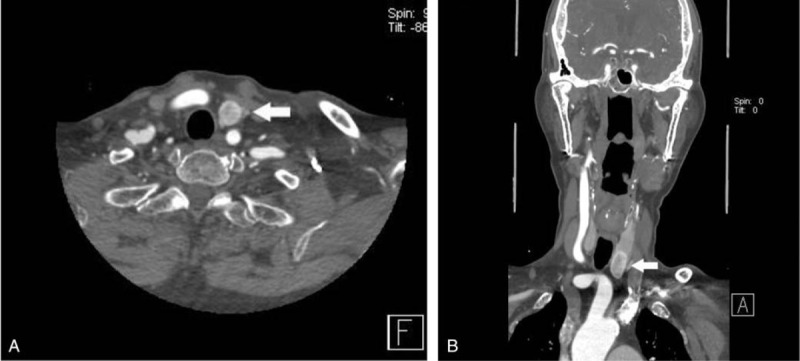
Axial (A) and coronal (B) CTA images demonstrating a 1.3 cm thyroid nodule within the left lobe of the thyroid gland (white arrow). This incidental finding was not initially reported on neck CTA.
